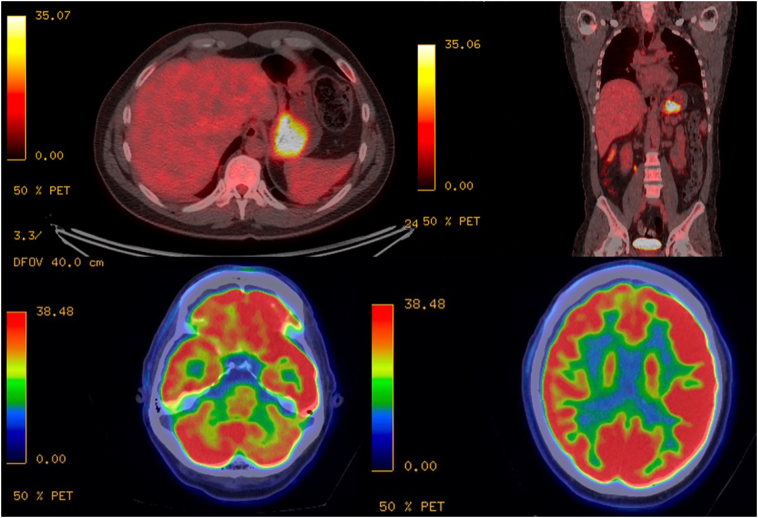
PET-CT: Above: Hypermetabolism thickening of gastric infiltrative aspect fundocorporal topography accompanied by perigastric adenopathies. Below: Brain hypometabolism, diffusely compromising the right cerebral hemisphere, frontal, occipital, parietal and temporal lobes, generating asymmetry with respect to the left side, accompanied by crossed cerebellar diaschisis with diffuse left cerebellar hypometabolism, diffuse right thalamic hypometabolism and relative sparing of the basal ganglia nuclei, some patches of mild hypometabolism in the frontal region.
